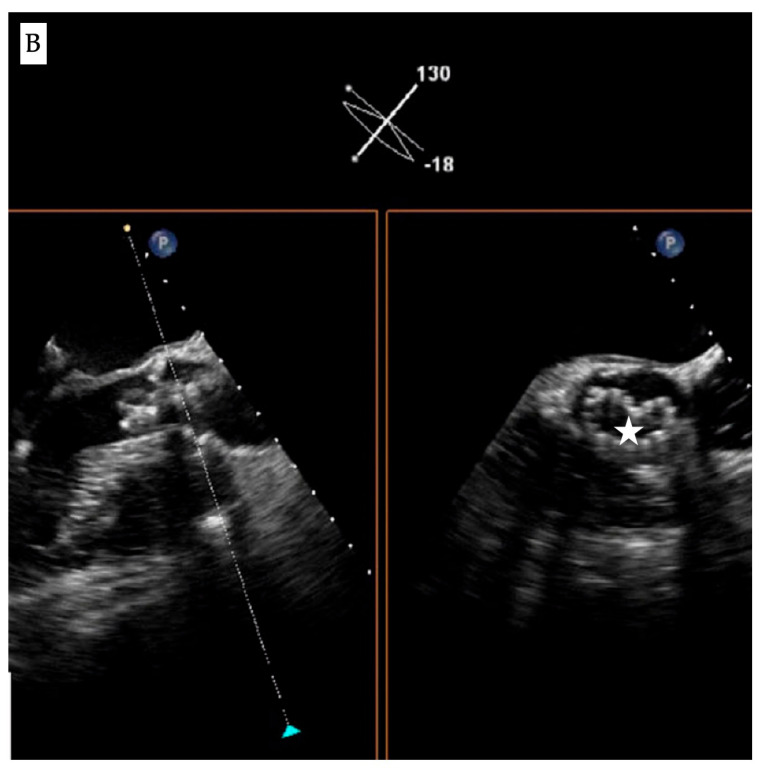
Fluoroscopic and echocardiographic appearance of TAVI infolding. (A) Visual clues for recognizing this complication include a dense vertical line across the valve frame (white arrow) and the inability to reach the intended valve expansion. (B) Short axis window in echocardiography revealing the “Pac man” sign (white star).
